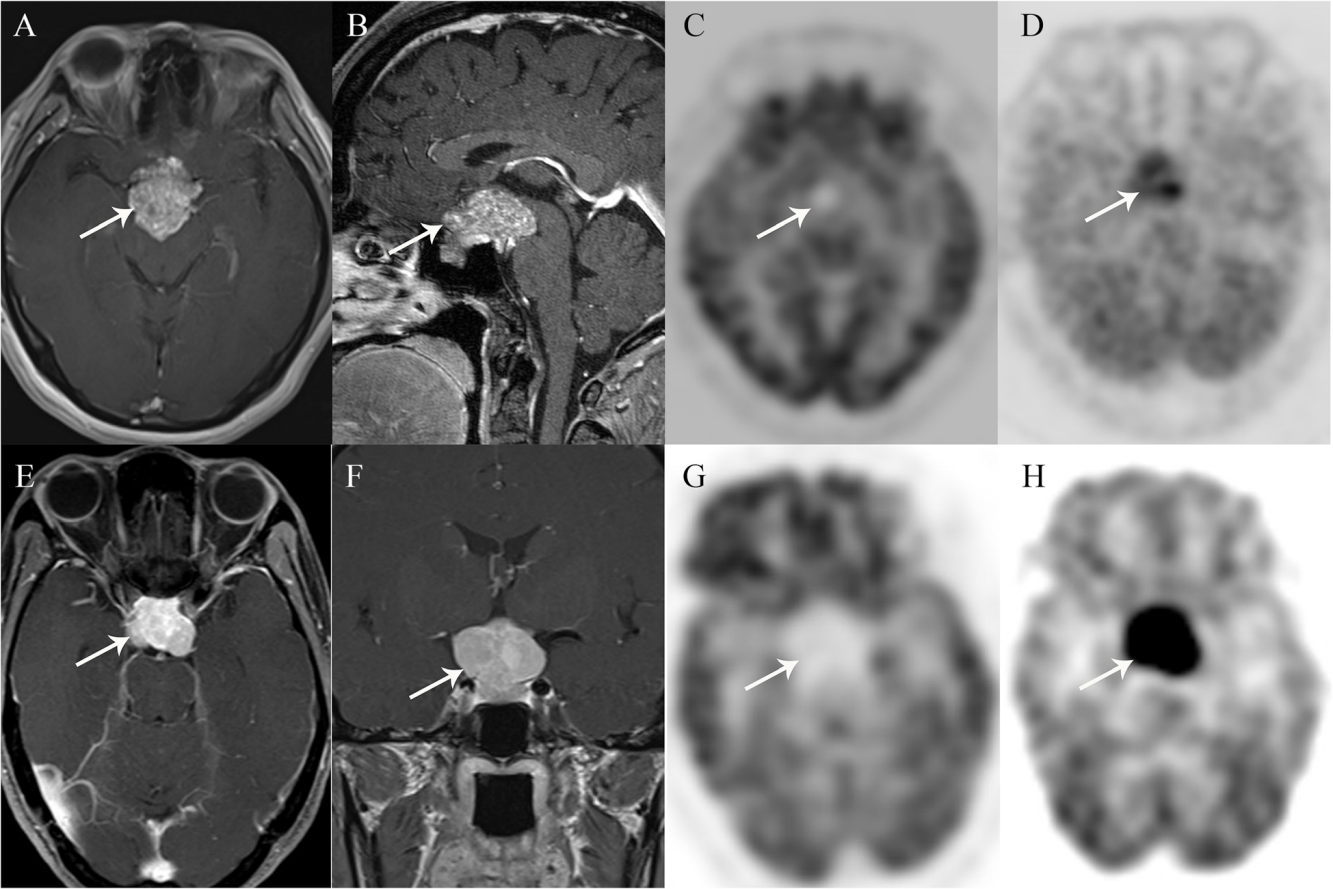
Imaging results in cases with NFPMA(A-D) and SSM(E-F). Trans-axial and sagittal contrast MRI images (a, b) showed the tumor (white arrows) had obvious enhancement. The pituitary gland was under the tumor intactly (white arrowheads) and the tumor was misdiagnosed as a pituitary macroadenoma on MRI images. Slight uptake of ^18^F-FDG (c) and high uptake of ^13^N-ammonia (d) on PET images. Trans-axial and coronal contrast MRI images (e, f) showed a marked enhancement (white arrows) mass mostly in the suprasellar region. The mass grew into the pituitary fossa and the pituitary gland was compressed. The lesion displayed obviously higher uptake of ^13^N-ammonia (h) compared with normal gray matter and low uptake of ^18^F-FDG (g)
